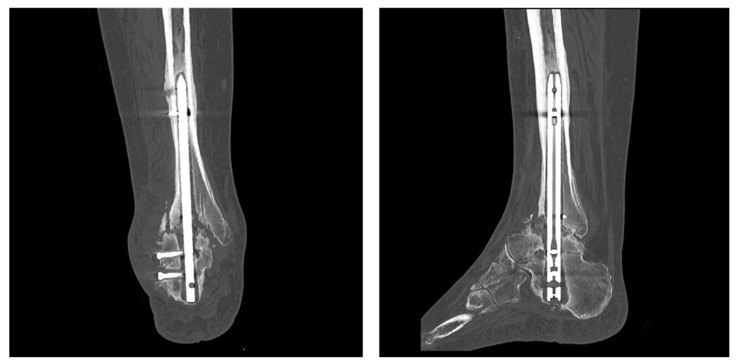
Anteroposterior (AP) and lateral computed tomography (CT) scans obtained 10 months after surgery, demonstrating limited osseous bridging with incomplete consolidation at the tibiotalar and subtalar fusion sites.
